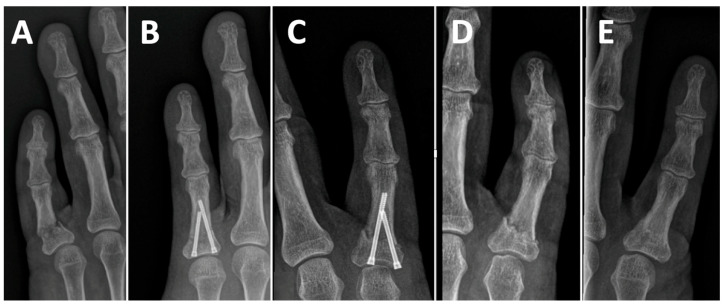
Radiographic sequence of a 60-year-old male patient with a base fracture of the proximal phalanx of the right little finger. (A). Anteroposterior radiograph at the time of trauma. (B). Postoperative radiograph following double-barrel antegrade intramedullary screw fixation, demonstrating adequate fracture reduction. (C). Follow-up radiograph showing fracture collapse and protrusion of the head of the shorter screw. (D). Radiograph after removal of the screws. (E). Final radiograph demonstrating fracture consolidation.
